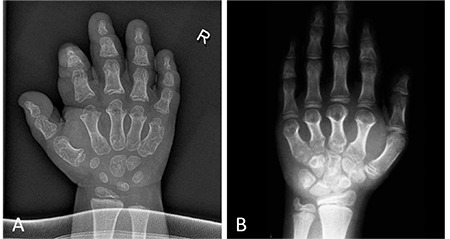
X-rays of the hands of patient 1 (A) and patient 2 (B). Note the severe brachydactyly with shortening of metacarpals and phalanges and cone-shaped epiphyses [Figure 2B reproduced with permission of Springer (17)]
